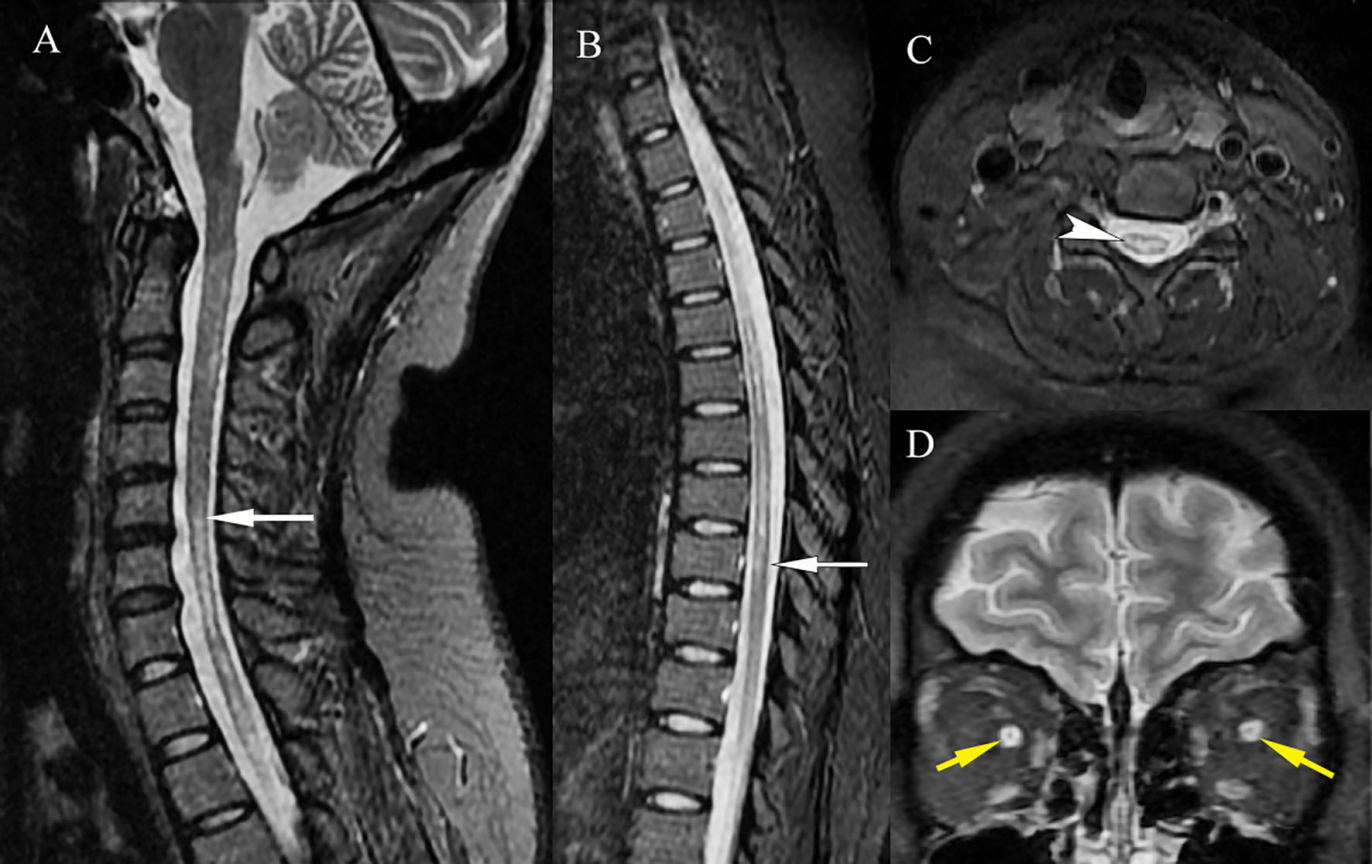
MRI of the NMOSD lesions. MRI of the cervical and thoracic spine showed a longitudinally extensive T2-hyperintense lesion [(A, B), white arrows], which involved the central portion of the cord [(C), white arrowhead]. A significant enhancement and thickening of the bilateral optic nerve sheaths were observed [(D), yellow arrows].
